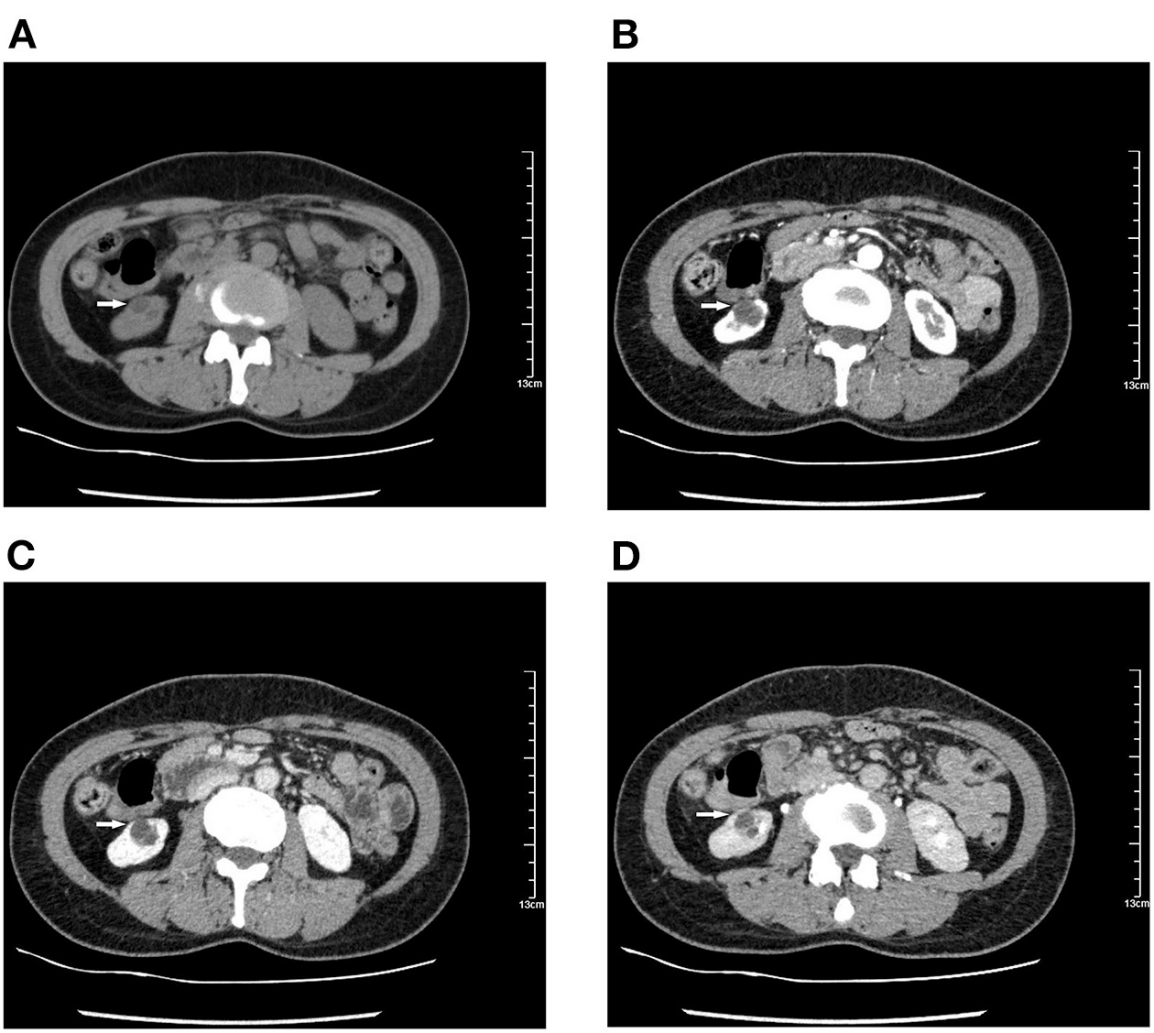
Computed tomography (CT) shows a hypodense mass of 1.8 × 1.5 × 1.4 cm in the lower pole of the right kidney. Contrast-enhanced CT scan shows a polycystic mass with slight enhancement of the wall and septa and no enhancement in the cystic part. The right lower pole of the kidney is partially depressed, and contrast-enhanced images show local thinning of the renal cortex during cortical enhancement. (A) Unenhanced images. (B–D) With contrast. (B) Renal cortical phase. (C) Renal corticomedullary phase. (D) Renal excretory phase.
